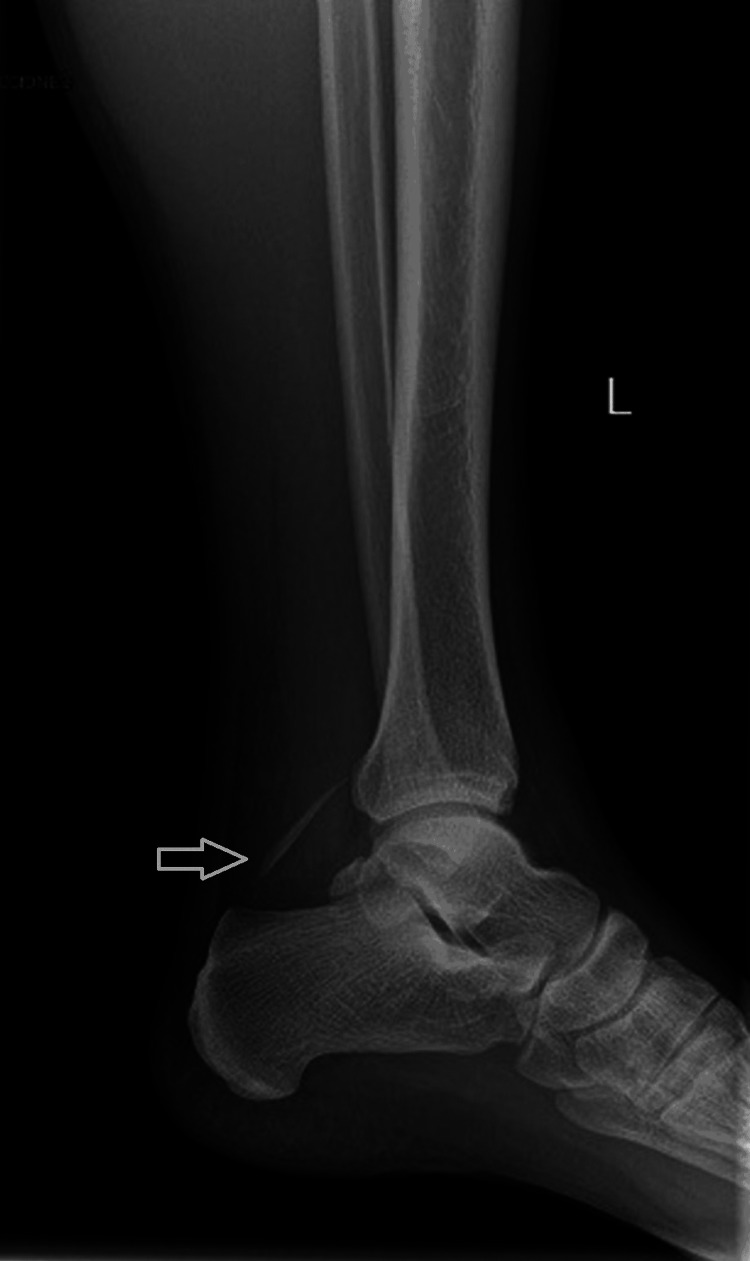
Lateral X-ray of the leg with a radiopaque object superior to the calcaneus marked by the arrow compatible with a stingray barb.
